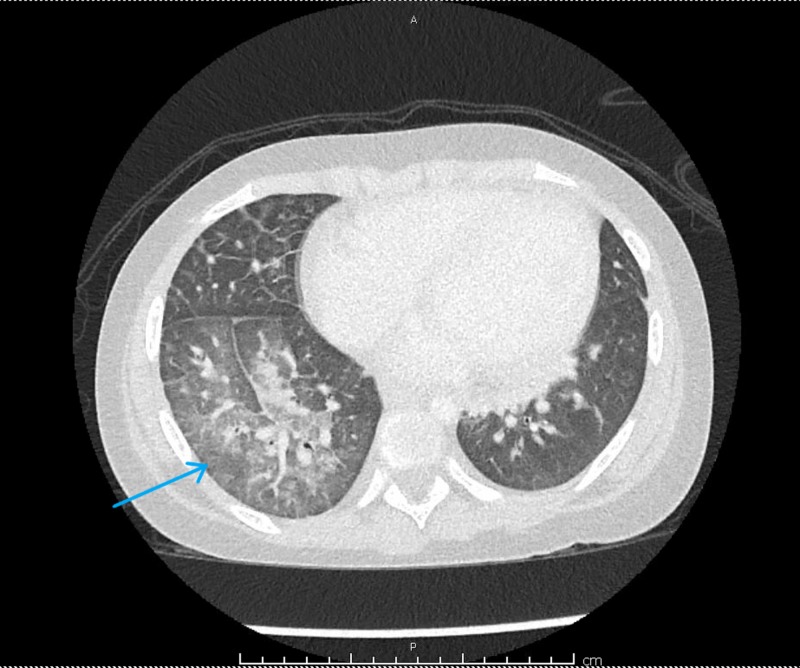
CTA chest Diffuse inflammatory changes with alveolar hemorrhage, as well as ground-glass opacities, noted in the right middle and lower lobes with left lower lobe atelectasis and hilar lymphadenopathy. CTA - Computed Tomography Angiogram
